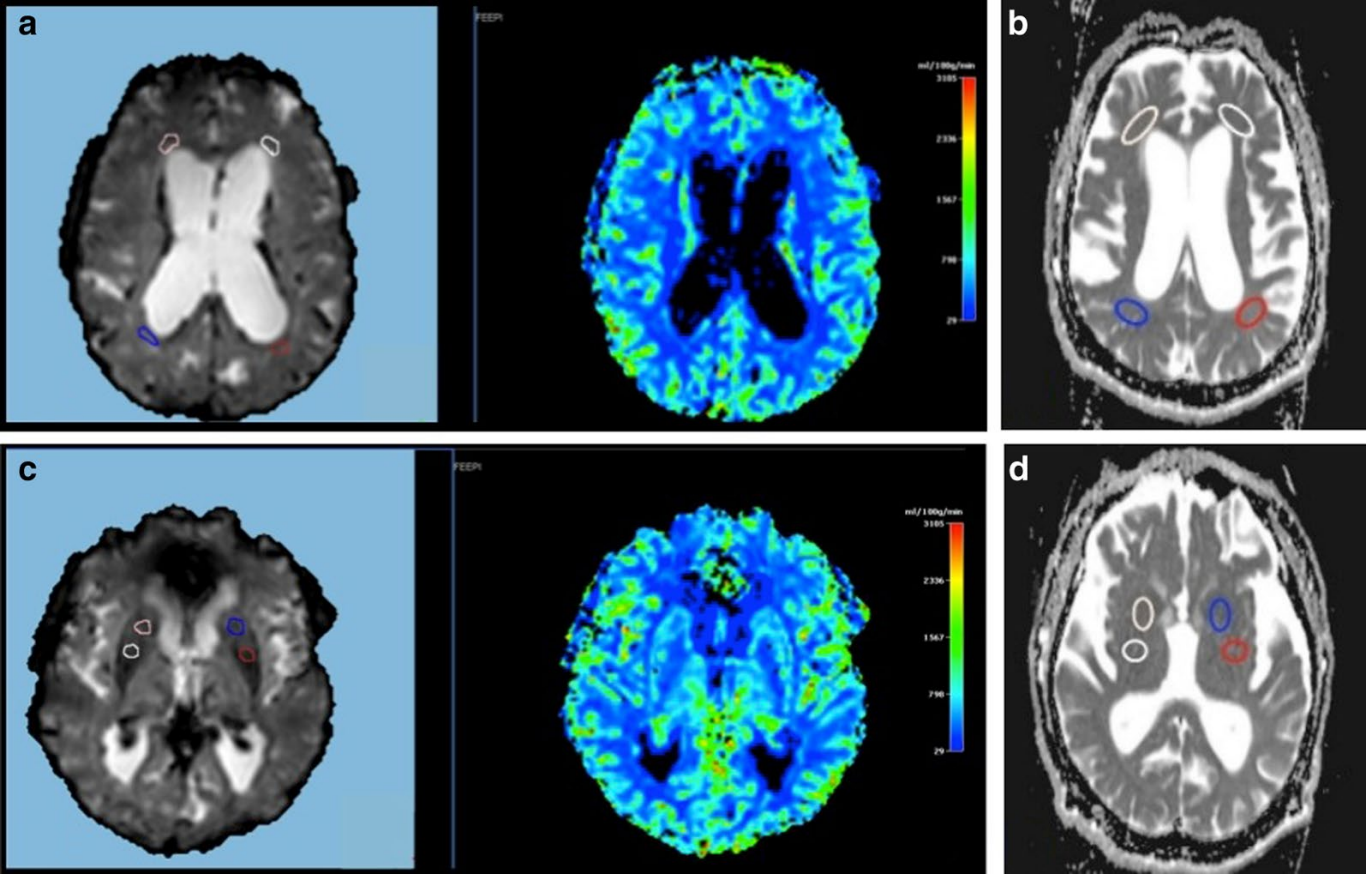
MR images of patient G. a, c Regions of interest (ROIs) positioned on perfusion maps in periventricular and basal ganglia region respectively. b, d ROIs positioned on ADC diffusion maps in periventricular and basal ganglia region respectively
